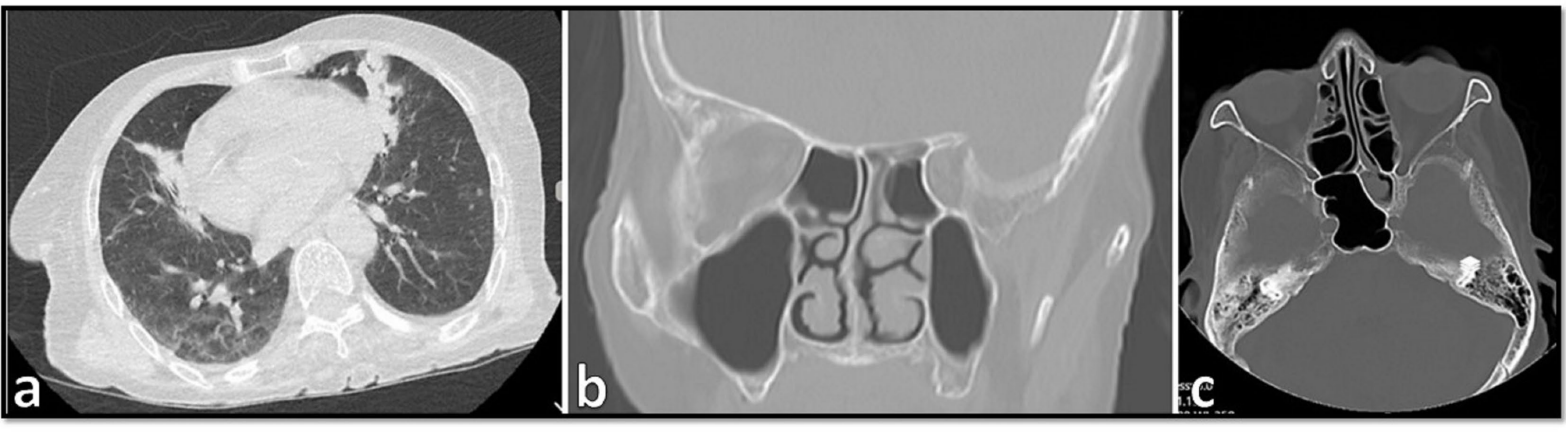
(A) Computed tomography scan of the chest in the case showing bilateral ground-glass opacities with a predominantly peripheral and multilobar location. (B,C) Orbital computed tomography scans of paranasal sinuses demonstrating opacification and air-fluid level in the left sphenoid sinus and some anterior ethmoid sinus air cells in right septal deviation to the right mucosal thickening in the left maxillary sinus.
